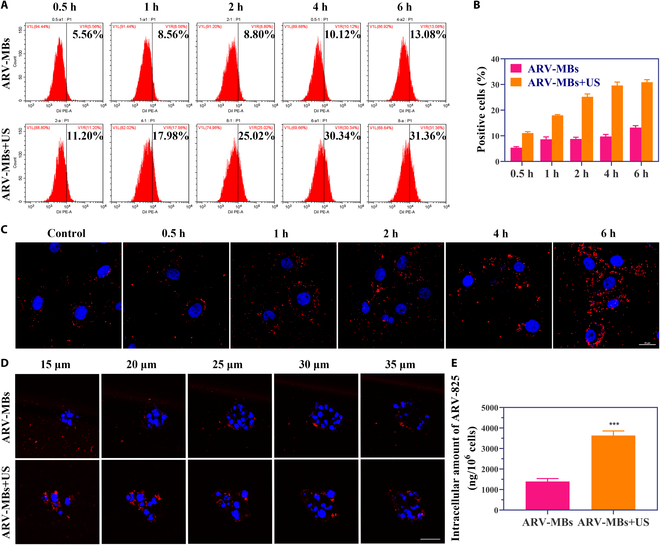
Qualitive cellular uptake assay. (A and B) Flow cytometry plots (A) and corresponding quantitative fluorescence intensity analyses (B; *n* = 3) of MDA-MB-231 cells treated with DiI-labeled microbubbles with or without ultrasound at different times. (C) Intracellular fluorescence of MDA-MB-231 cells incubated with DiI-labeled microbubbles for different times observed by CLSM. Scale bar, 25 μm. (D) Fluorescence distribution of 3D tumor spheroids incubated with ARV-MBs with or without ultrasound observed by CLSM. Scale bar, 50 μm. (E) Quantitative analysis of intracellular amount of ARV-825 in MDA-MB-231 cells treated with ARV-MBs in the presence or absence of ultrasound as determined by RP-HPLC (*n* = 3, P* < 0.001).
